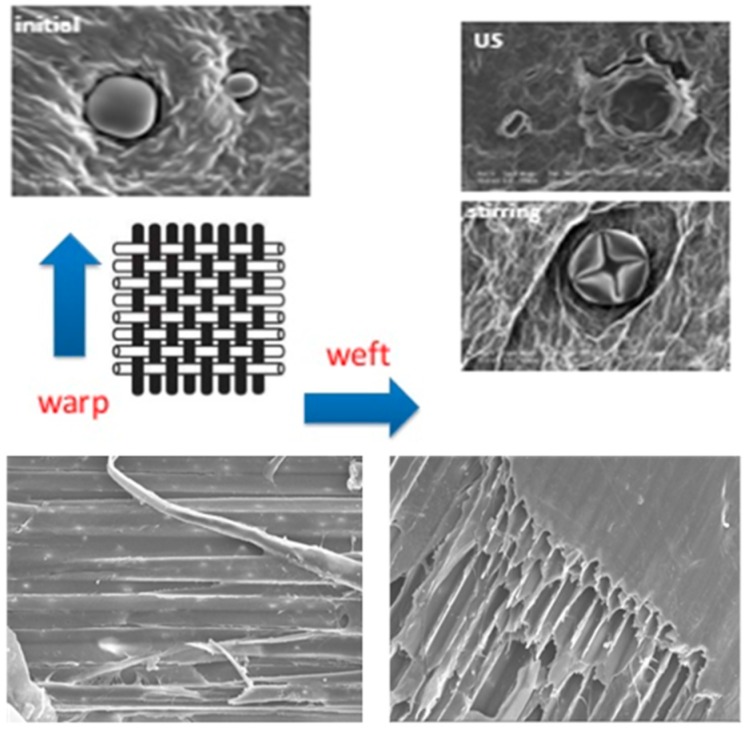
Dismantling biomass structure under hydrodynamic cavitation (HC) and ultrasound (US), like a fabric where ‘warp’ and ‘weft’ (from the authors’ laboratory).
